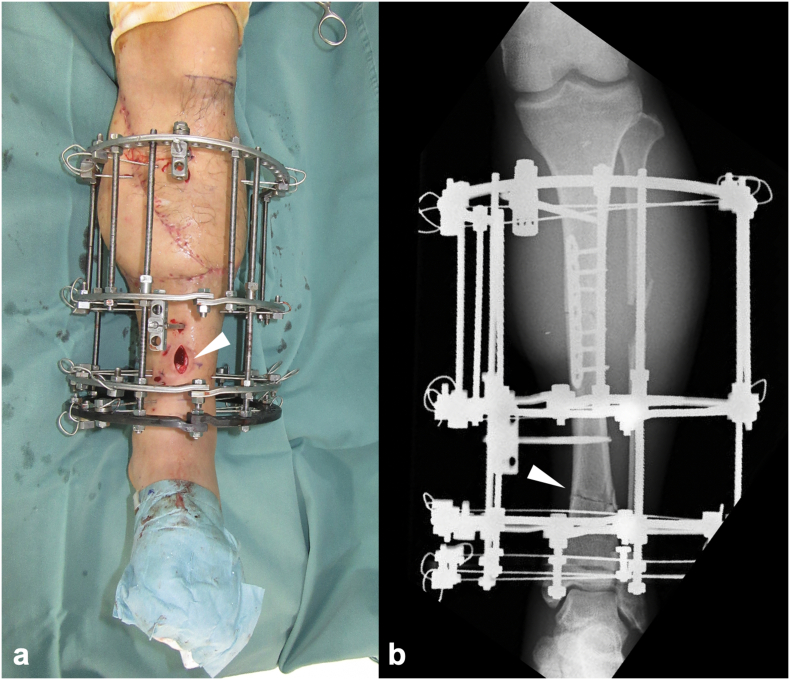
Findings following the application of the Ilizarov external fixator. (a) Intraoperative photograph showing the osteotomy site through a small incision (*(white arrowhead*). (b) Anteroposterior plain radiograph showing the osteotomy at the distal diaphysis of the tibia (*(white arrowhead*).
